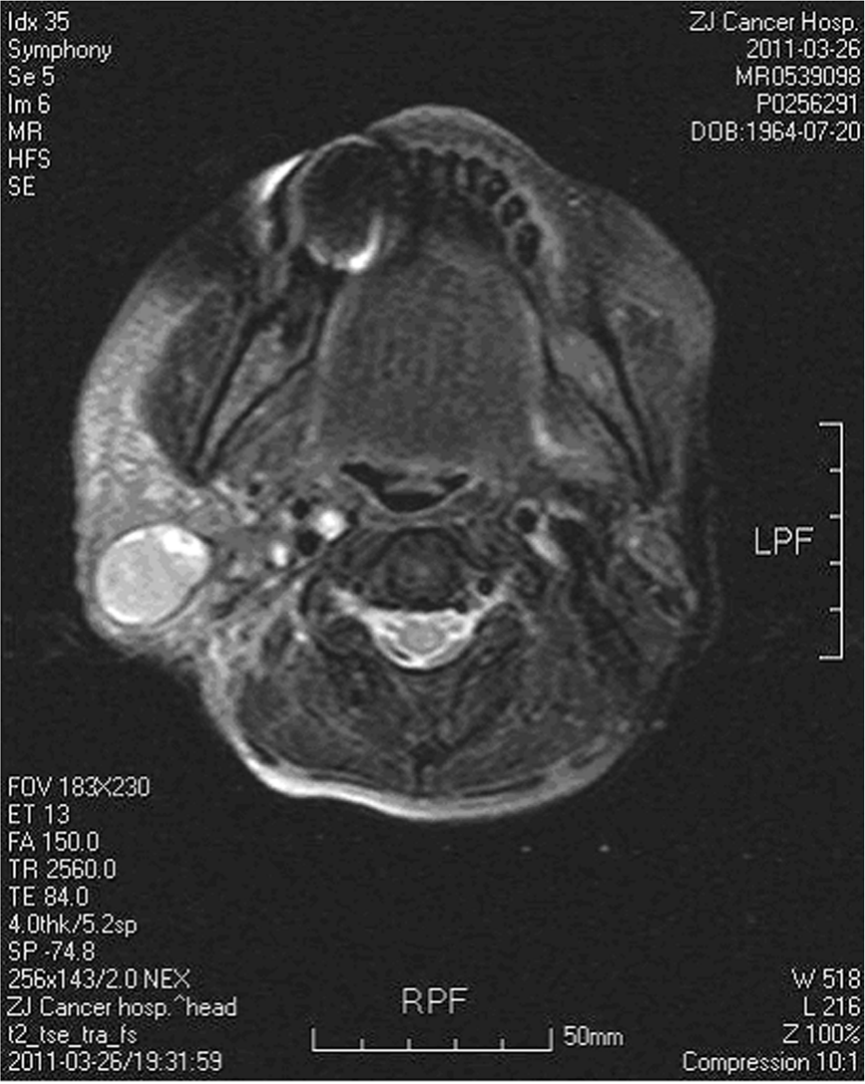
MRI image of uncontrolled parotid metastasis of nasopharyngeal carcinoma after radiotherapy. Multiple swollen lymph nodes were observed in bilateral carotid sheath and pharyngeal lymph node area.
